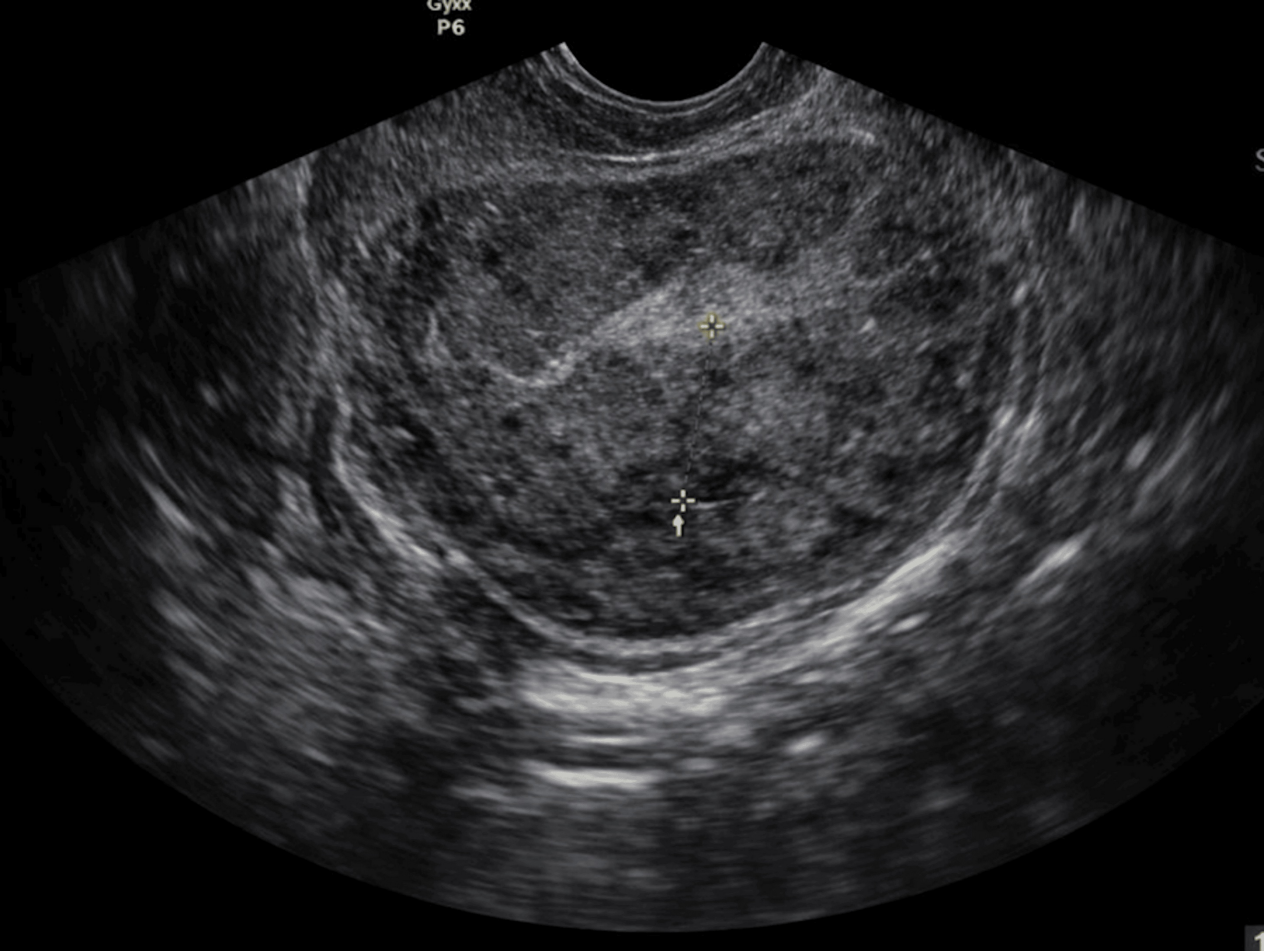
Preoperative Transvaginal Ultrasound The transvaginal ultrasound image demonstrates a markedly globular and bulky uterus that has lost its typical pear-shaped configuration, a finding highly characteristic of diffuse adenomyosis. Within the uterine wall, the myometrium exhibits a heteroechoic and mottled appearance, indicating an irregular internal texture rather than the smooth, uniform gray seen in a healthy organ. Subtle linear striations and fan-shaped shadowing are visible, representing the acoustic reflection of ectopic endometrial tissue and small cystic spaces embedded deep within the muscular layer. The junctional zone between the endometrial lining and the myometrium appears thickened and poorly defined, which further supports the diagnosis.
